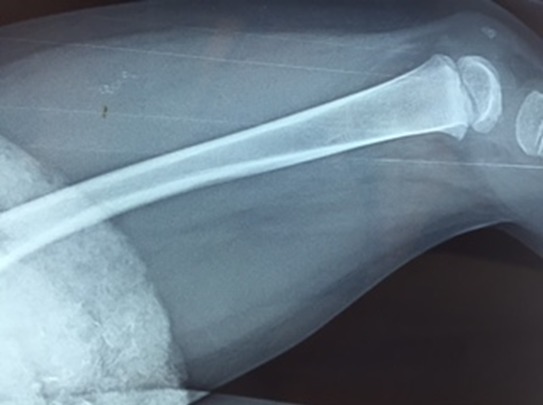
Radiographie standard de profil montrant la guérison de la lésion après traitement
